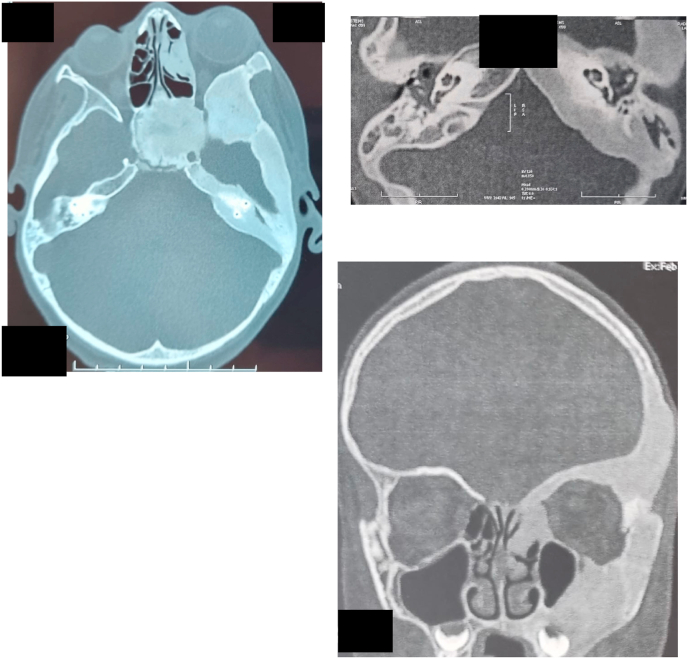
Coronal CT scan shows involvement of the petrous part of the left temporal bone. The superior semicircular canal is surrounded by dysplasia.
